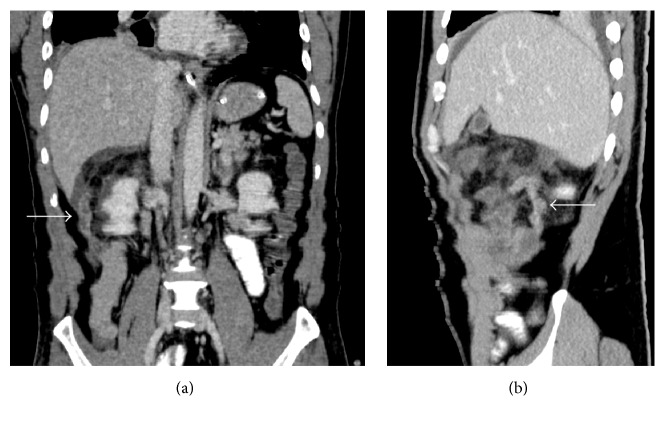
(a) Coronal image and (b) sagittal image of the contrasted CT scan showing the subhepatic appendix (white solid arrow).
